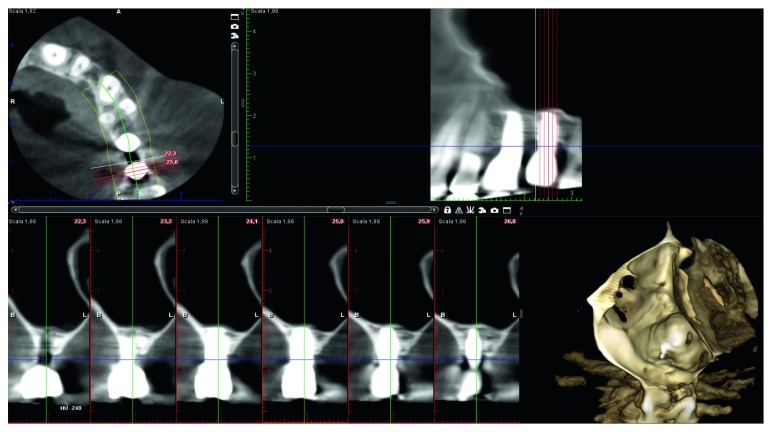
Implant site #26 CT scan at 4 years.
